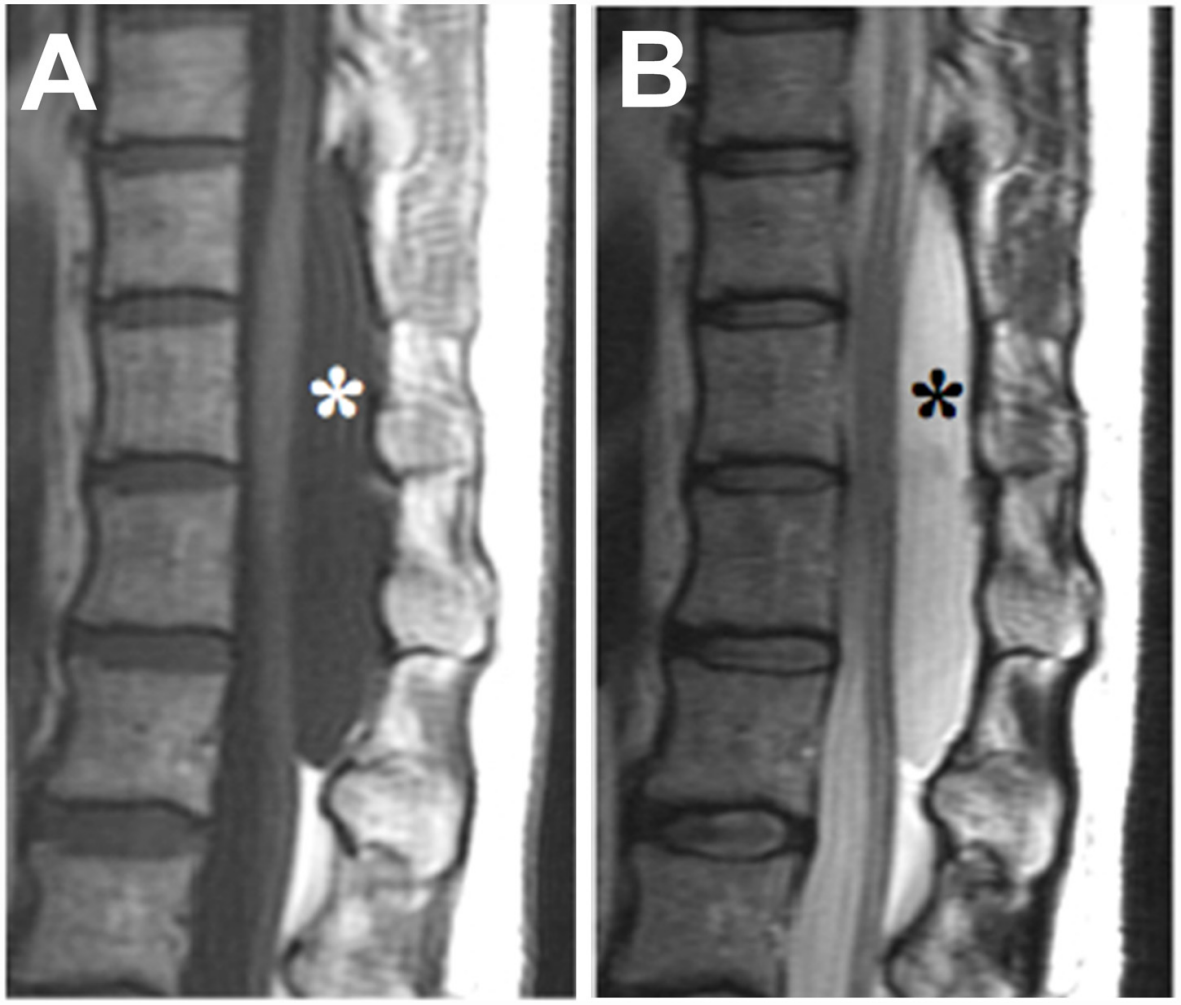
Spinal extradural arachnoid cyst. T1- (A) and T2- (B) weighted sagittal plane images of MRI scan. Subject P5. There is a single cyst (asterisk marks) dorsal to the spinal cord at the thoracolumbar spine.
